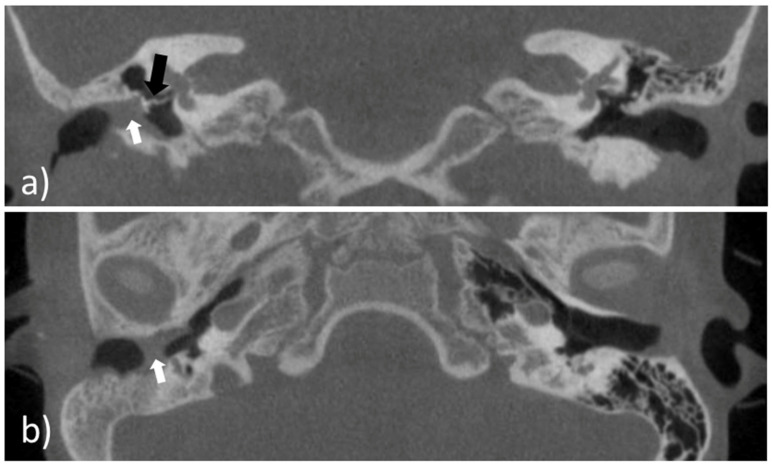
Cone beam computed tomography imaging of the temporal bone of the patient. (a) Coronal view of the external ear canal with the white arrowhead pointing to the restenosis; an aerated middle ear space and a middle ear partial ossicular replacement prosthesis (black arrowhead); (b) axial view of the external ear canal with the partial restenosis (white arrowhead).
